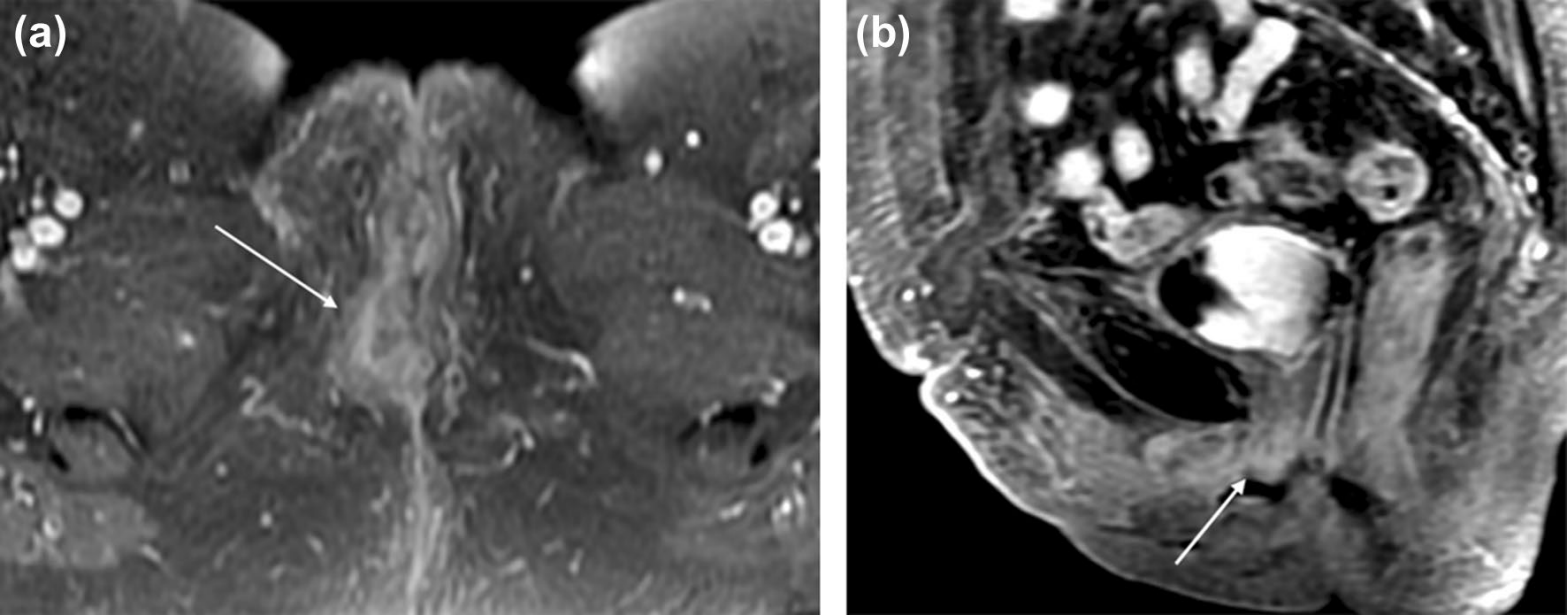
69-year-old woman with FIGO stage 2 squamous cell carcinoma of the vulva. a Axial T1-weighted fat saturation with contrast MR image of the pelvis at the level of the vaginal introitus demonstrates irregular thickening in the right labia (arrow). b Sagittal T1-weighted fat-saturated MR image with contrast demonstrates enhancing soft tissue surrounding the distal urethra (arrow), a feature of FIGO 2 stage tumor. Cystoscopy confirmed urethral involvement by tumor with meatal stenosis
